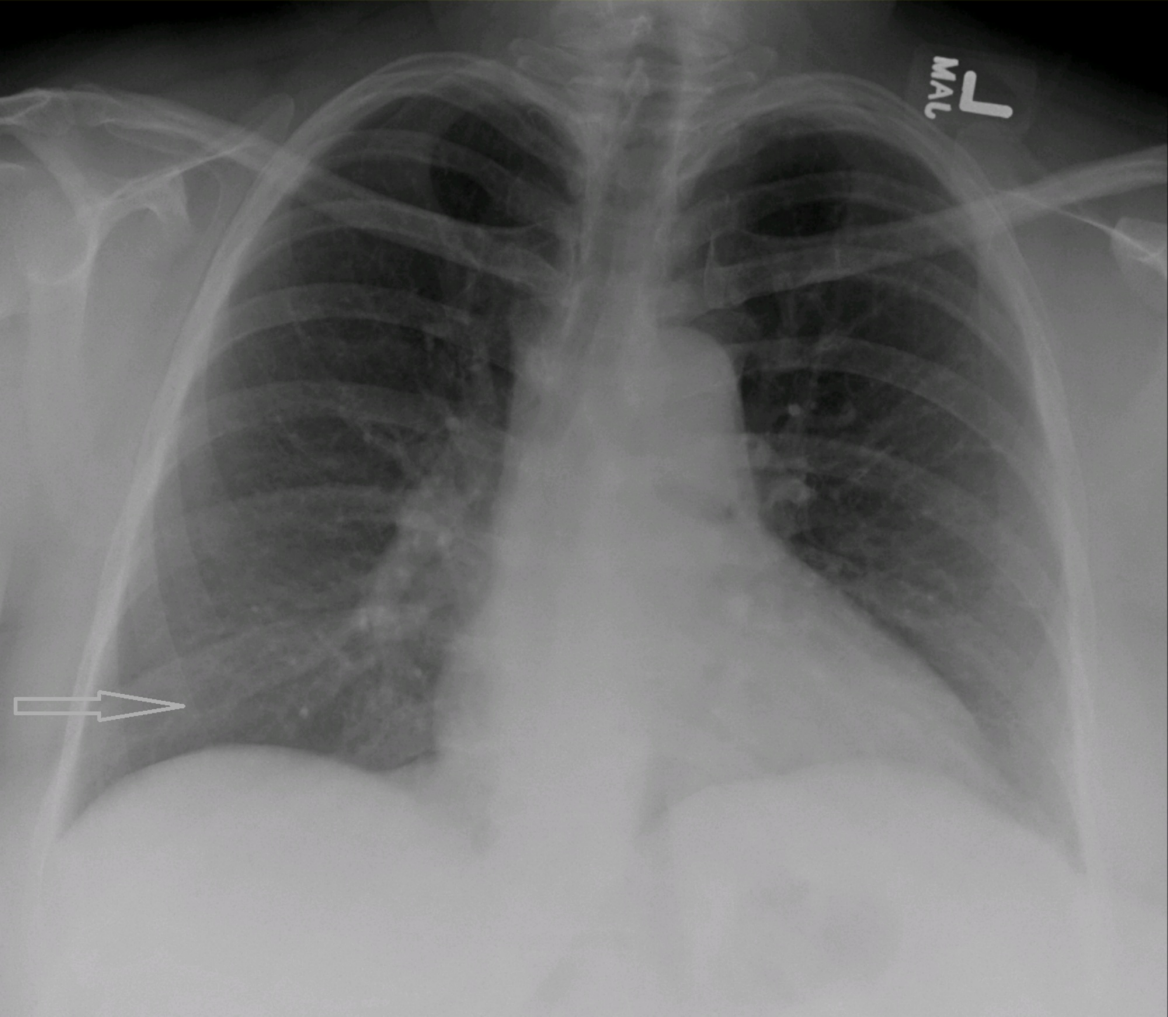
Chest X-ray showing the resolution of the right-sided pleural effusion (arrow)
